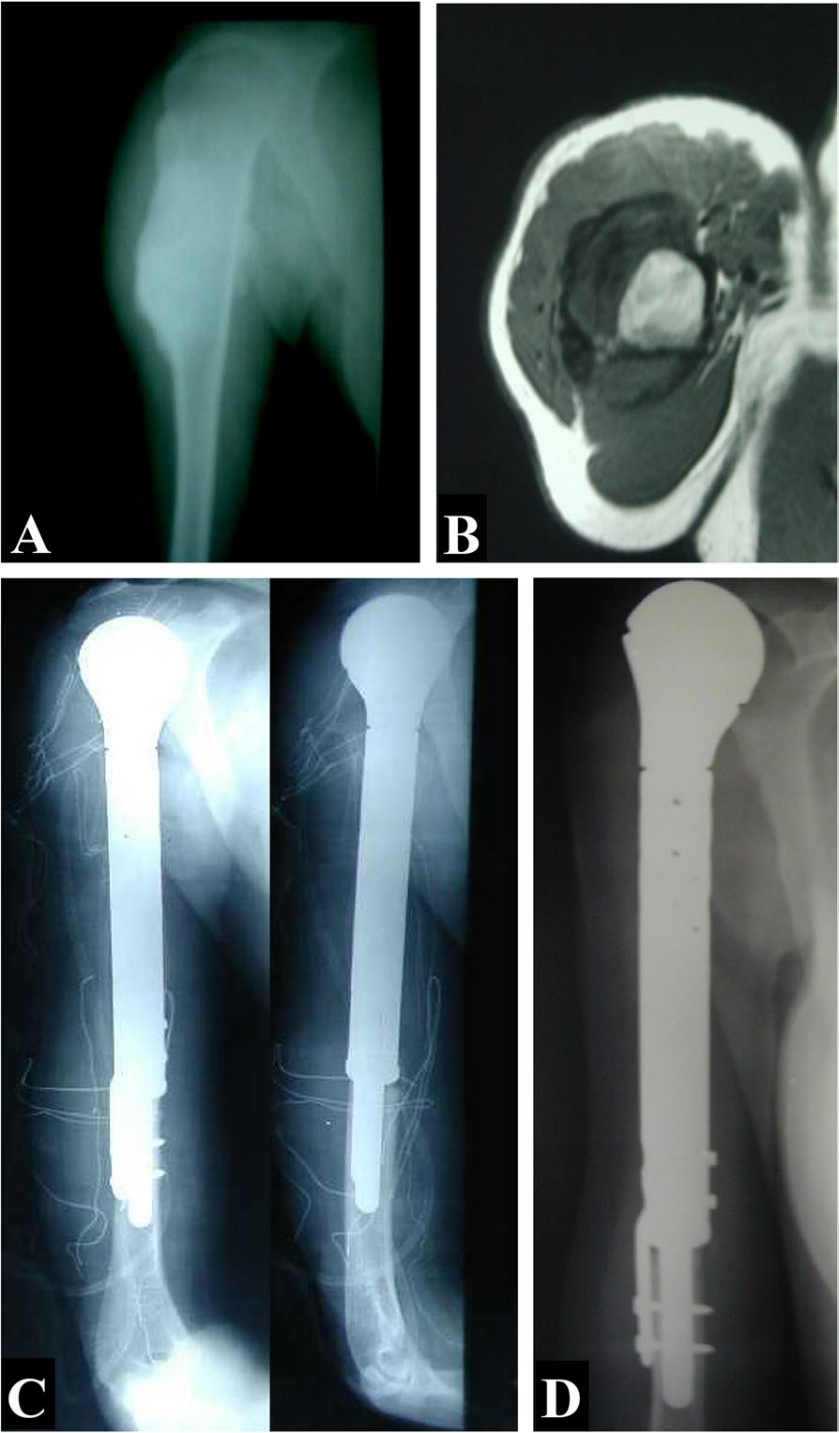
A 24-year-old female patient with osteosarcoma of the proximal humerus had undergone tumor resection and reconstruction with endoprosthesis. A Plain X-ray of the shoulder, anteroposterior view. B MRI axial view. C Postoperative X-rays, anteroposterior and lateral views. D Two-year follow-up X-rays, anteroposterior view
